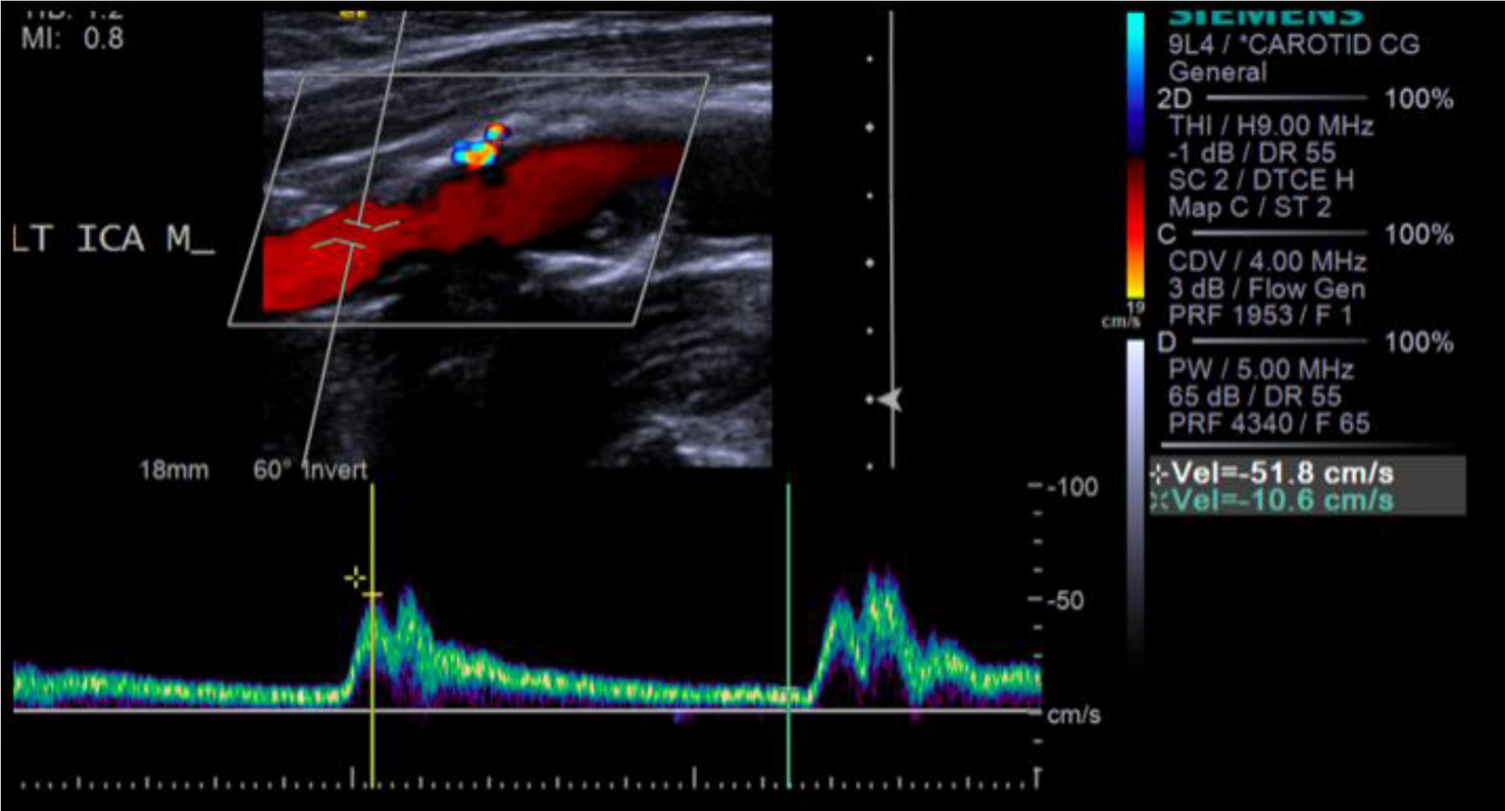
85-year-old male patient who presented with acute right-sided weakness. Carotid duplex ultrasonography left ICA: irregular echogenic plaque at the origin of the left ICA resulting in 0–29% stenosis. ICA, internal carotid artery.
